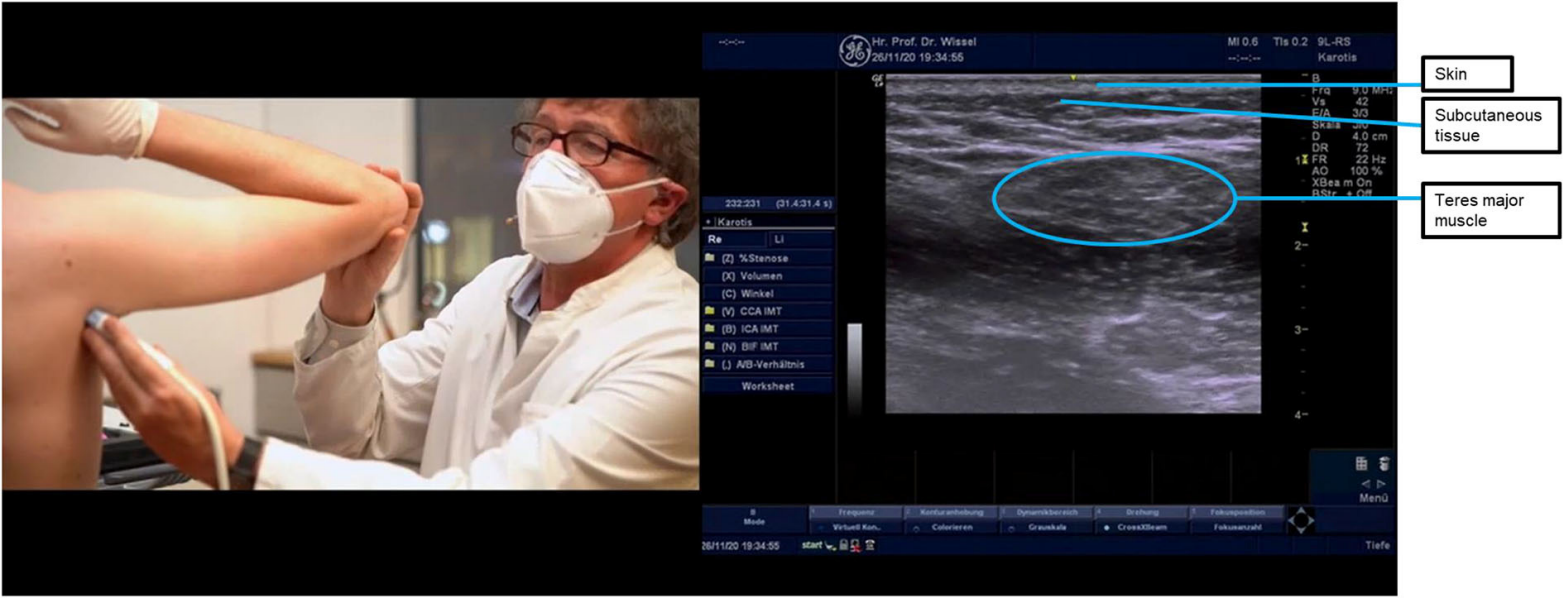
Ultrasound probe placement and image of the teres major muscle. The round muscle in the middle of the ultrasound image is the teres major muscle. The ultrasound probe can be turned through 90 degrees to help identification, with the teres major muscle having a striped appearance when the ultrasound probe is placed longitudinally.
